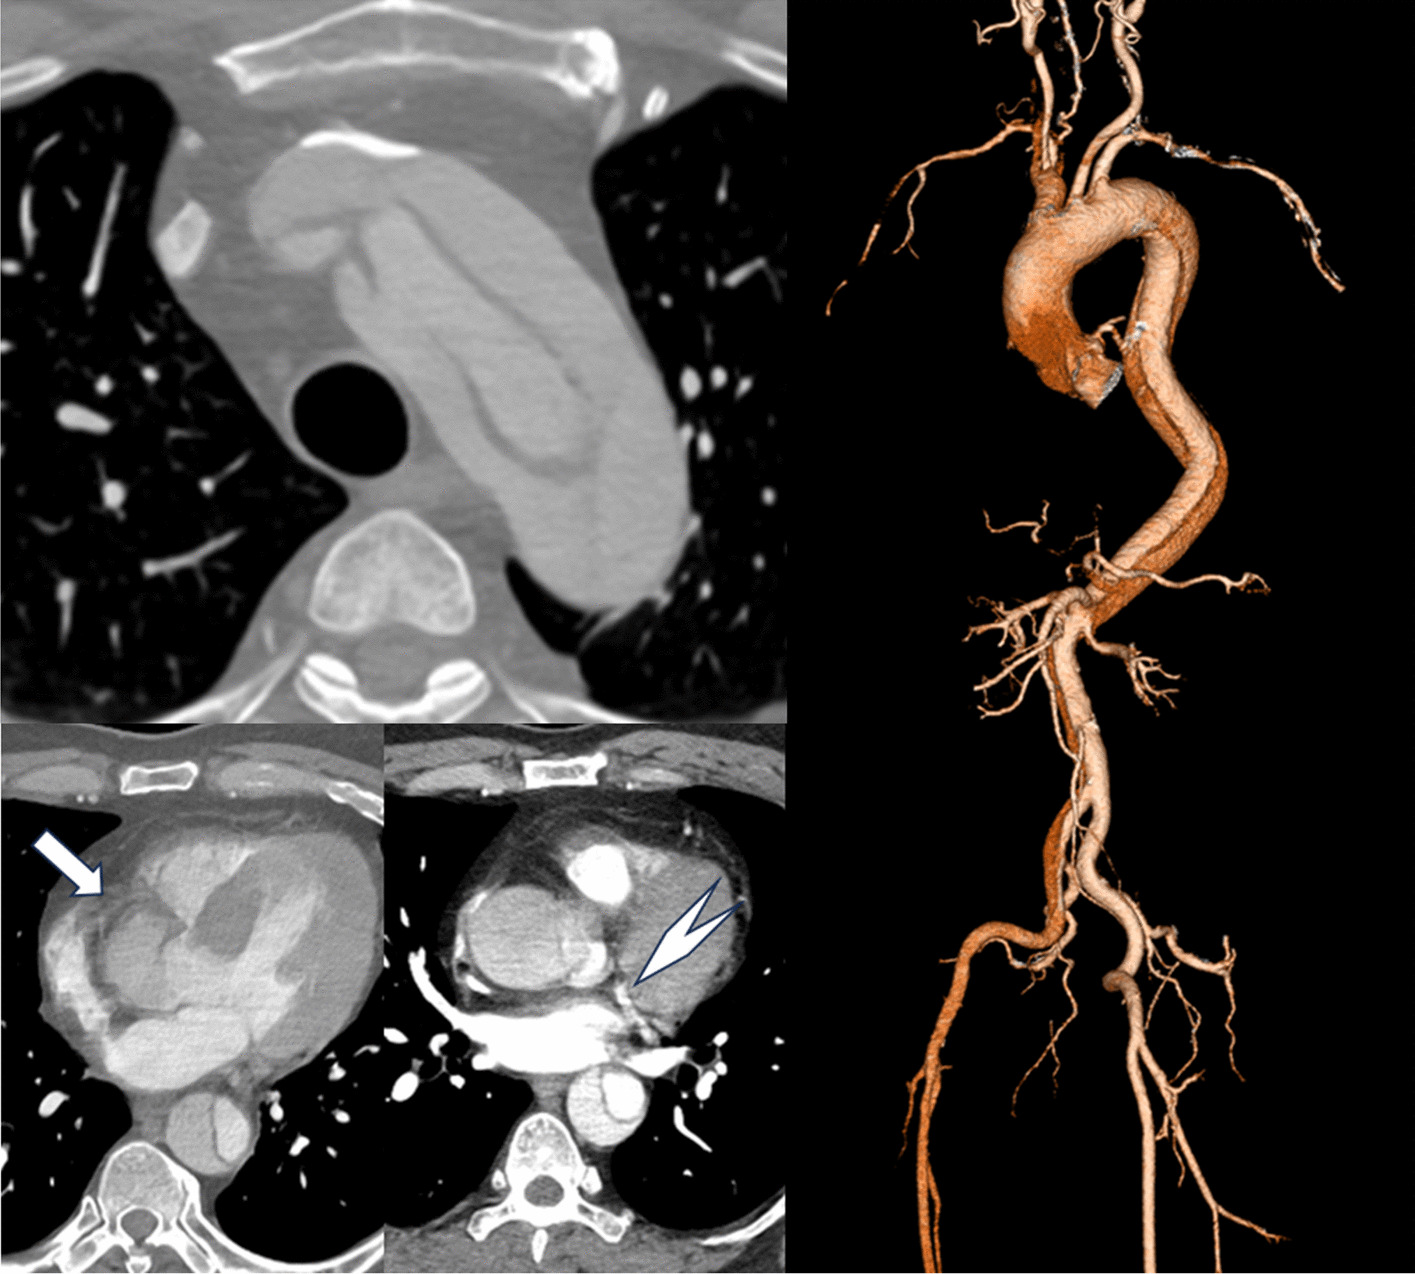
Three-dimensional computed tomography angiography (CTA) on admission. CTA revealed aortic dissection ranging from the aortic root to the iliac arteries. The enhancement of the right coronary artery (arrow) was weaker than that of the left coronary artery (arrowhead)
